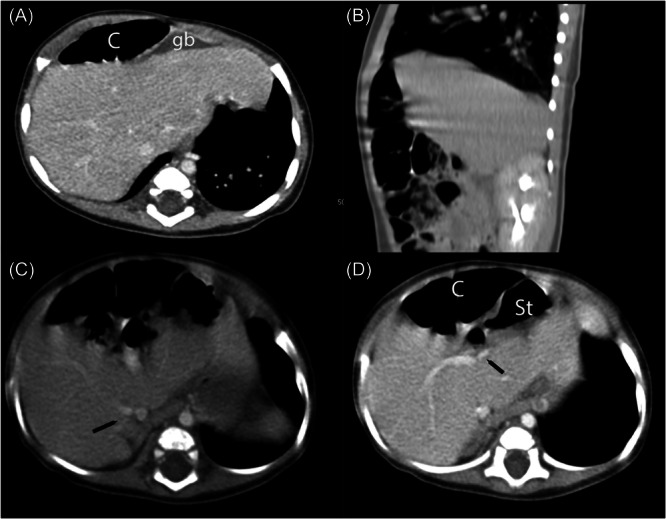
Contrast‐enhanced abdominal CT at age 4.5 months. (A) Axial image through the upper liver shows unusual position of the GB positioned to the left of midline and at the anterior surface of the liver. (B) Sagittal image demonstrates colonic interposition between the diaphragm and the liver in the right upper quadrant. (C) Axial image through the lower liver shows an accessory inferior right hepatic vein (*black arrow*), a common anatomic variant in the normal population. (D) Axial image through the mid liver shows branching of the main portal vein, with a patent but diminutive left portal vein (*black arrow*). C, colon; CT, computerized tomography; GB, gallbladder.
